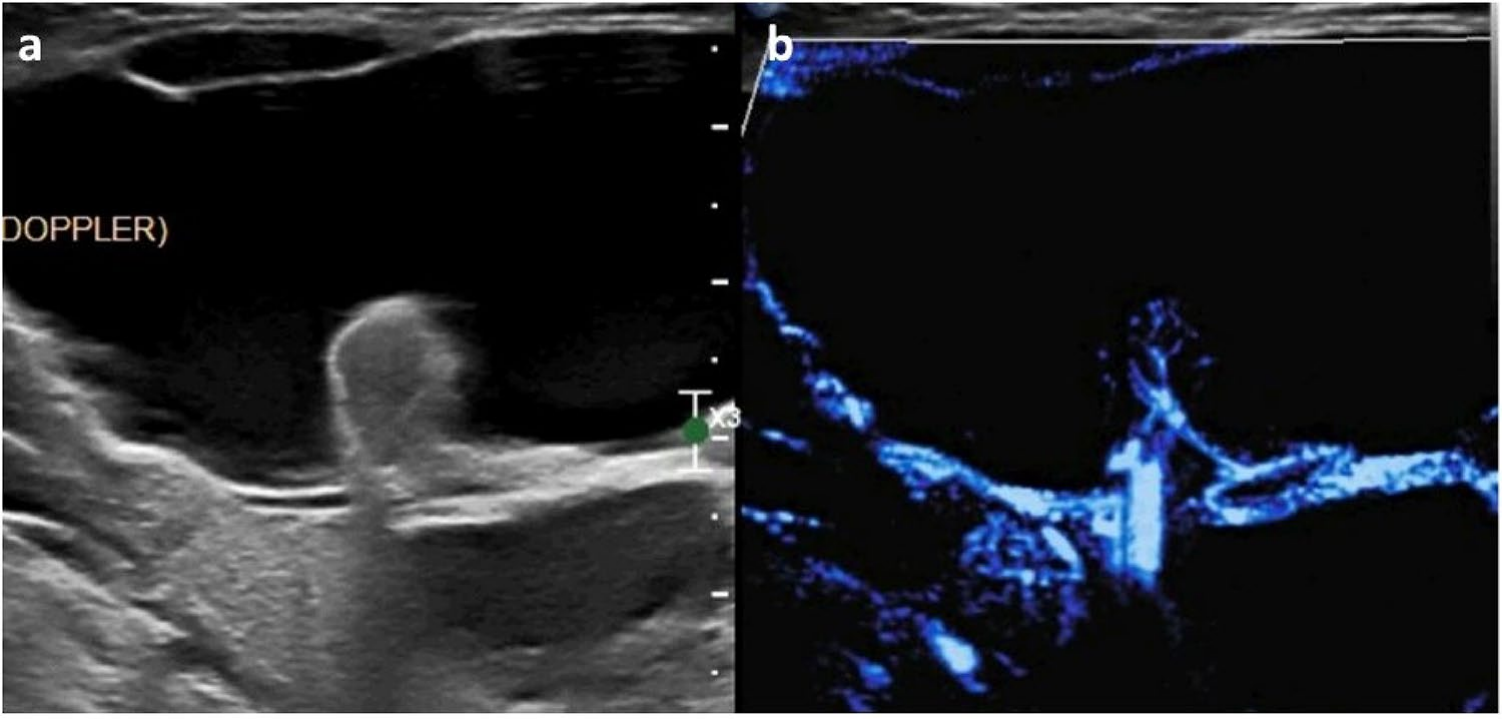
12-year-old boy. MVI as part of ultrasound for characterization of a neck mass that presented with left sided facial swelling. a Grayscale ultrasound demonstrated a large, predominantly cystic lesion with a solid central region, concerning for a solid nodule. b MVI showed hilar vessels, characteristic of a lymph node, most in keeping with a lymphatic malformation with a central lymph node
